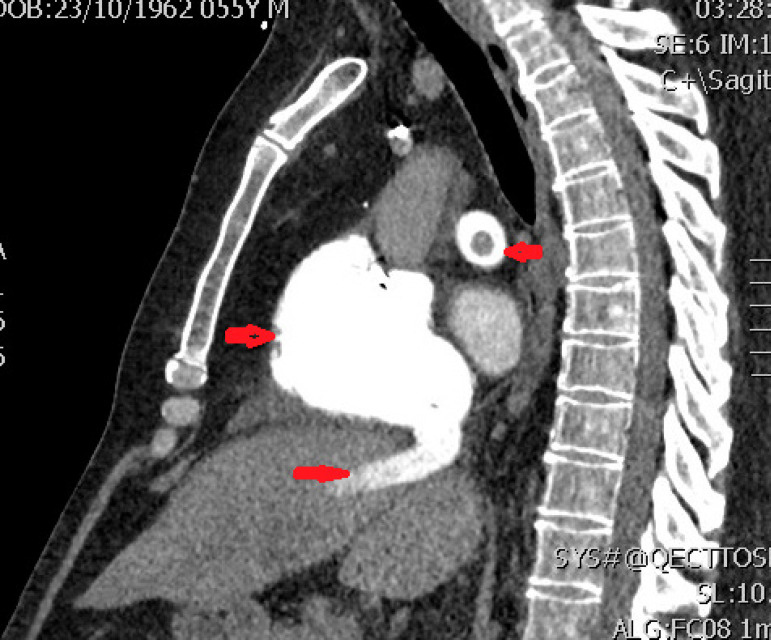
Computed tomography of pulmonary artery (sagittal view). Arrows show
